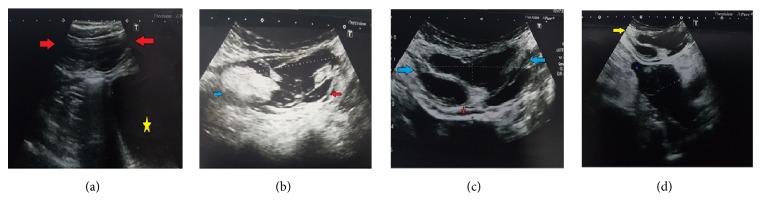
(a) B mode ultrasonographic image shows the SRSH. Increased muscle size with an ovoid fusiform border (red arrows) is seen. The SRSH is unilateral and does not dissect the fascial planes. Normal bladder is shown with yellow star (Type 1). (b) B mode ultrasonographic image shows that the SRSH is intramuscular but with blood between the muscle and the transversalis fascia, bilaterally. No blood was observed in the prevesical space. Complicated part of the SRSH is shown with blue arrow. Blood between the transversalis fascia and the muscle is shown with a red arrow (Type 2). (c) In the ultrasonographic image, the SRSH affects the muscle (blue arrows), and the blood is seen between the transversalis fascia and the muscle (red star) (Type 3). (d) In the ultrasonographic image, the SRSH affects the muscle (yellow arrow), and the blood is seen in the peritoneum and perivascular space (purple star) (Type 3).
